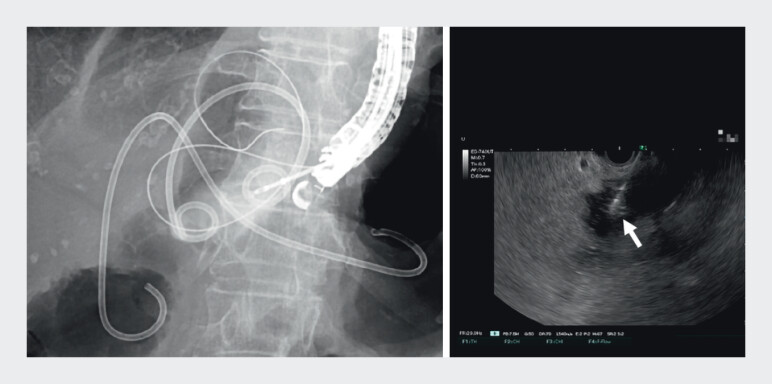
Fluoroscopic and endoscopic ultrasonography (EUS) images showing the migrated stent being grasped under EUS guidance using a sheath (Endosheather, PIOLAX) and biopsy forceps.
